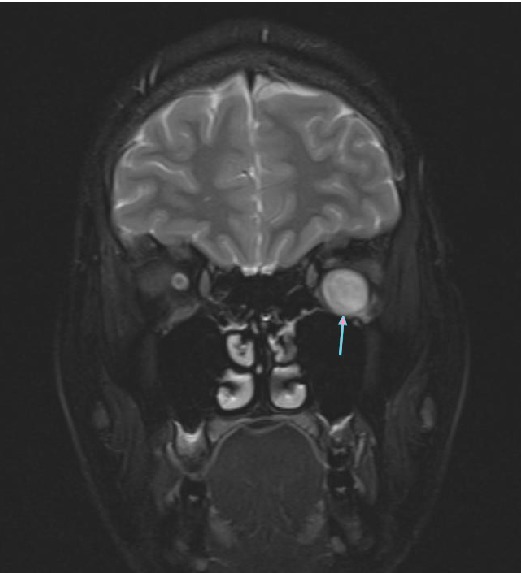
Coronal T2 weighted MR image showing hyperintense haematoma (arrow).
